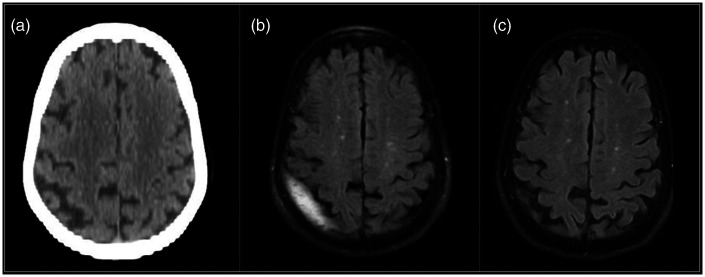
Seventy-eight year old (a) with a negative outside hospital CT following trauma but (b) a small subdural collection on MRI. (c) Collection resolved on imaging two months following MMA embolization.
